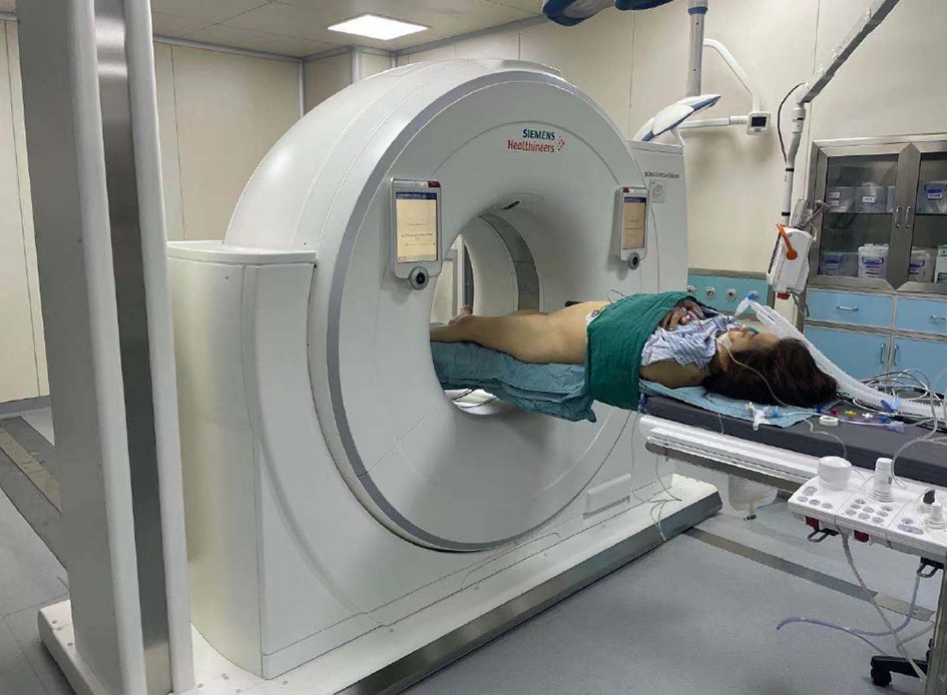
Standard posture of the patients with sacroiliac screw fixation navigated by intraoperative CT.
